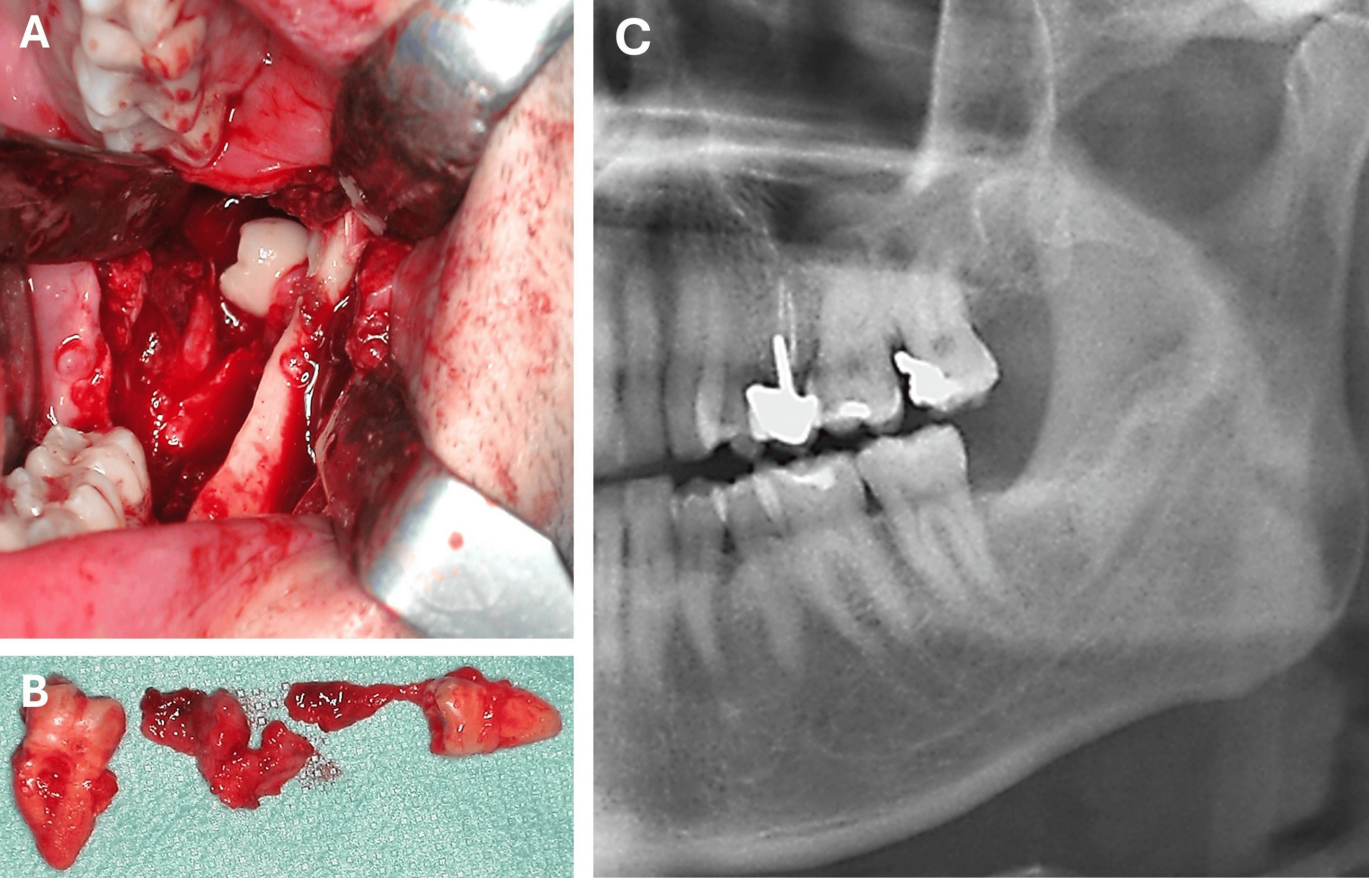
Intra-operative images and post-operative panoramic radiograph. (A) Intraoral approach to the impacted fourth molars and an adjacent dentigerous cyst. (B) Extraction of the left third and fourth molars, together with enucleation of the associated cyst. (C) Five-year follow-up panoramic radiograph.
